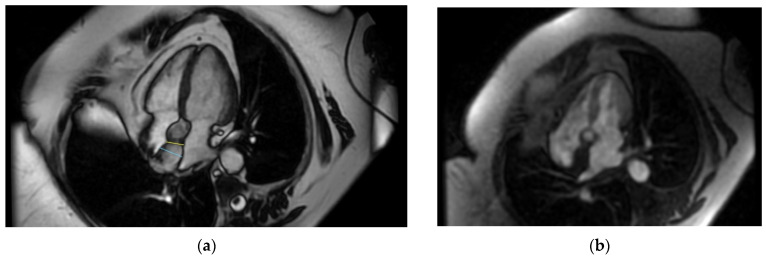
Cardiac MRI. (a) Four-chamber-view, steady-state-free precession image revealing interatrial septal hypertrophy—25 mm with “chemical shift”; (b) perfusion image—no caption of contrast at first-pass contrast.
